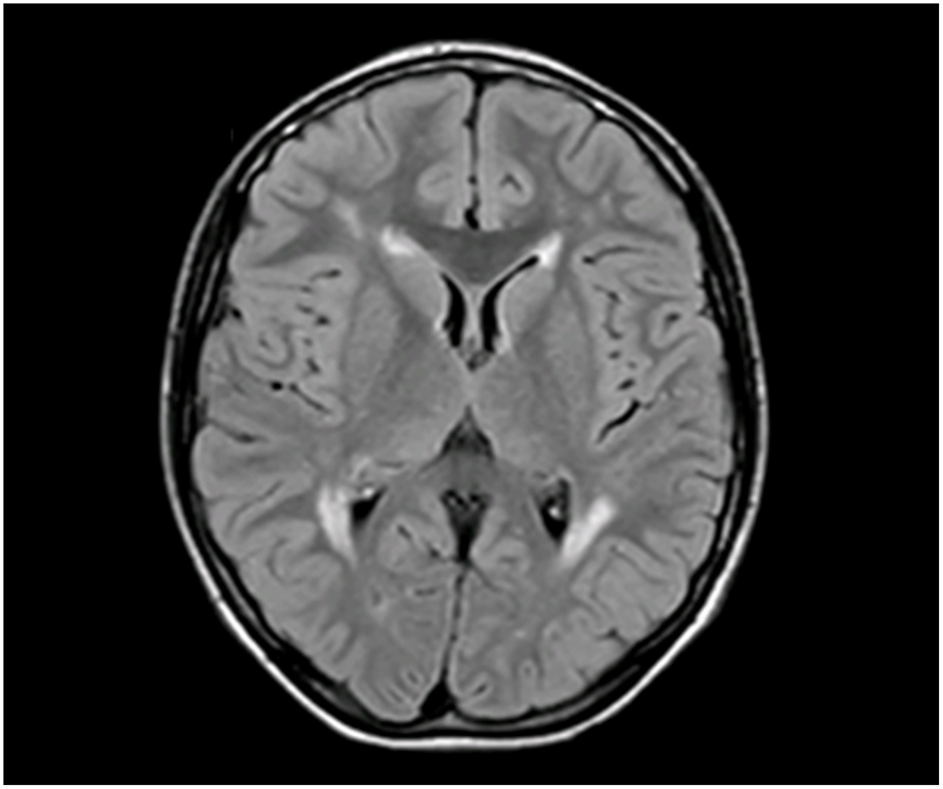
Brain MRI at 3 years, axial view: supratentorial white matter increased the T2 signal in the periventricular and frontal areas.
